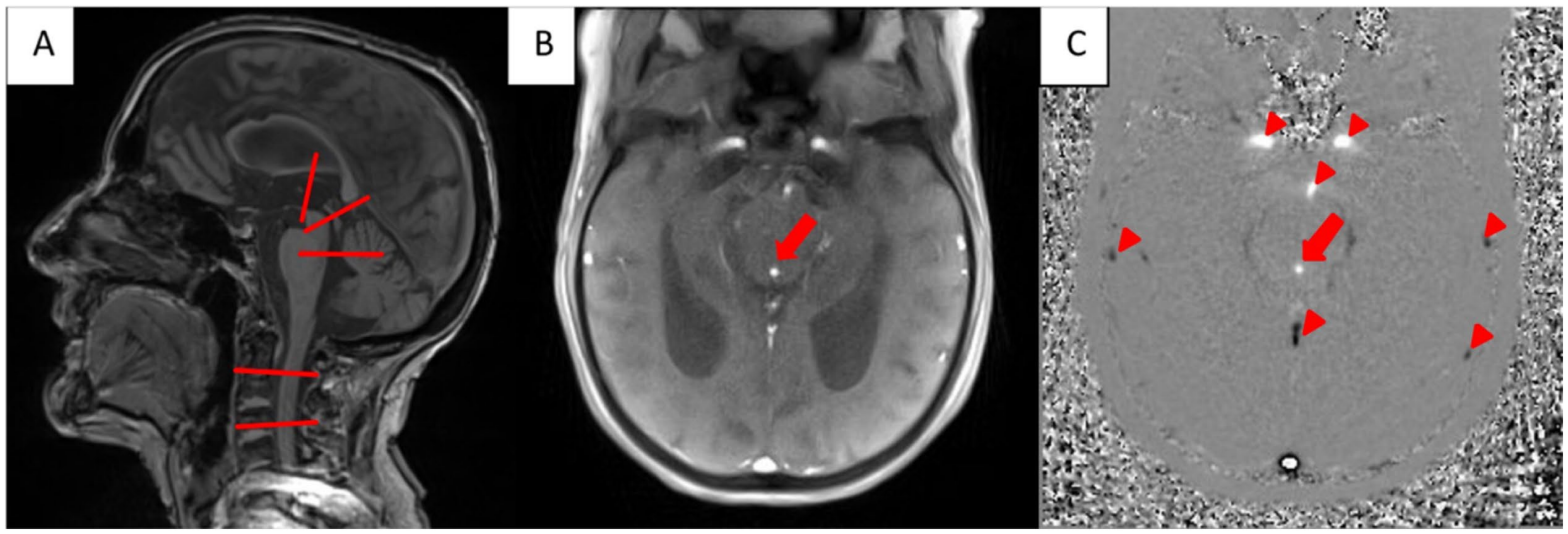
(A) T1-weighted MRI in sagittal plane. The red lines mark the ROIs for the CSF-flow measurements: third ventricle, aquaeductus mesencephalic, fourth ventricle, extracranial: subarachnoid space (second and fourth cervical vertebra). (B) Anatomical magnitude real time phase contrast MRI in a transversal plane showing CSF-flow in the aquaeductus mesencephalic (red arrow). (C) Corresponding phase contrast image with cranially directed CSF-flow (blue arrow). Note that in the phase contrast image cranially directed flow is visualized hyperintense and caudally directed flow hypointense (Arrowheads mark the carotid arteries and the basilar artery (hyperintense) and draining veins/sinus).
